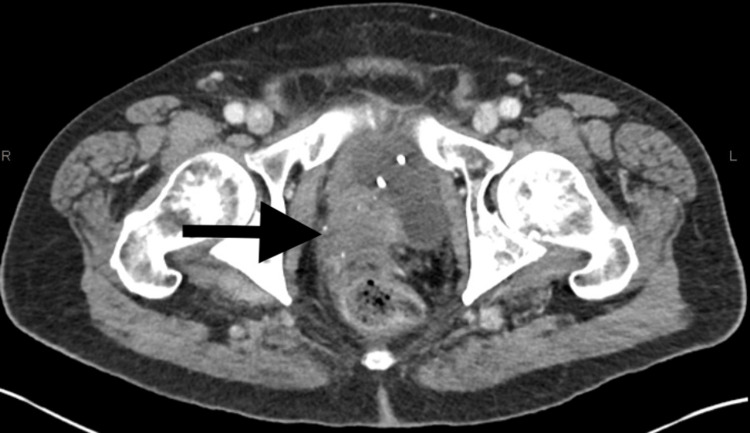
CT abdomen and pelvis axial view (post treatment)
